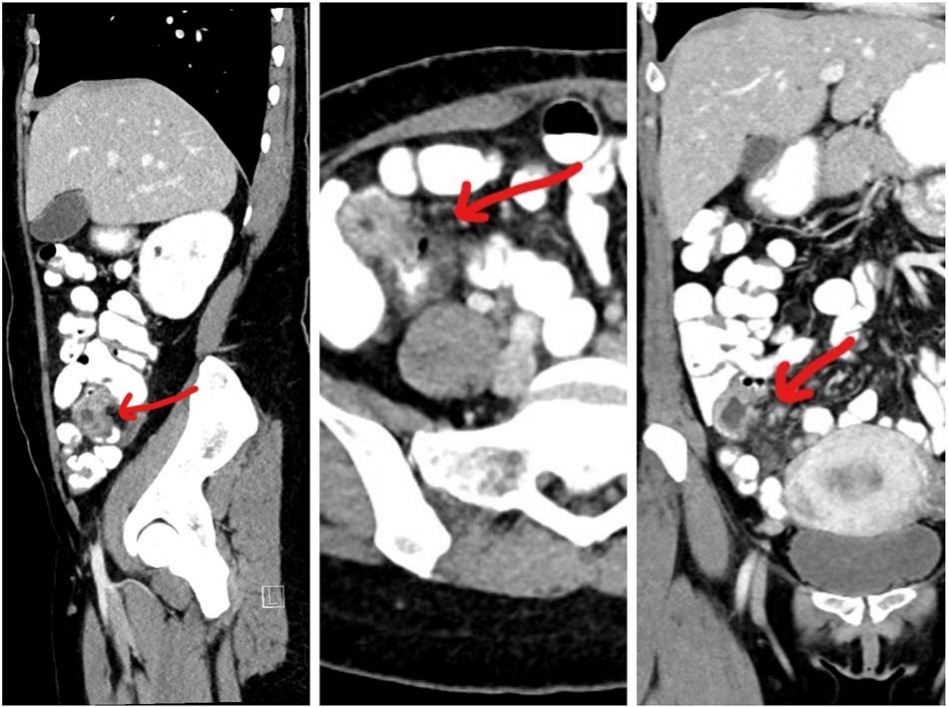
CT images showing the lesion in the right iliac fossa.
